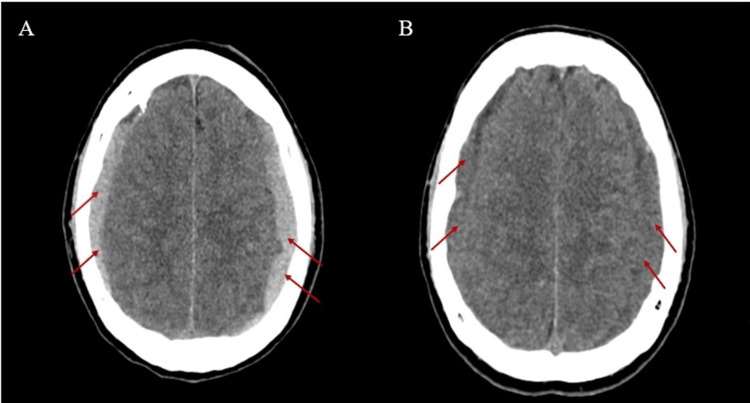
First and second brain CT scan The initial brain CT scan shows a bilateral subacute subdural hematoma (red arrows) (A). The second scan shows a bilateral chronic subdural hematoma (red arrows) (B). CT: computed tomography
